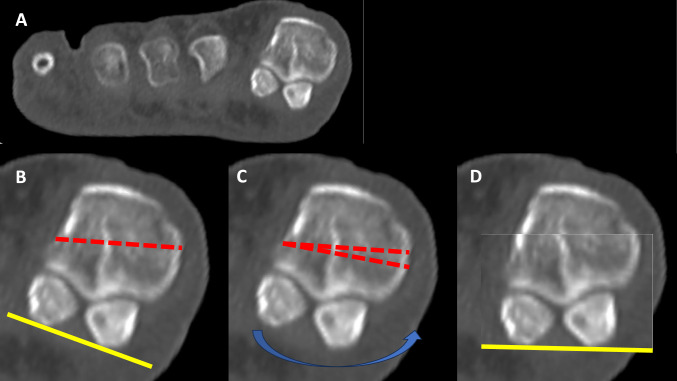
In A, coronal computed tomography scan of the metatarsal heads including sesamoids with some degree of pronation. In B example of a transverse (longitudinal) cut performed during a Scarf Akin osteotomy. In C example of re-cut of the plantar fragment performed in order to remove a wedge and to derotate the first metatarsal head (D)
